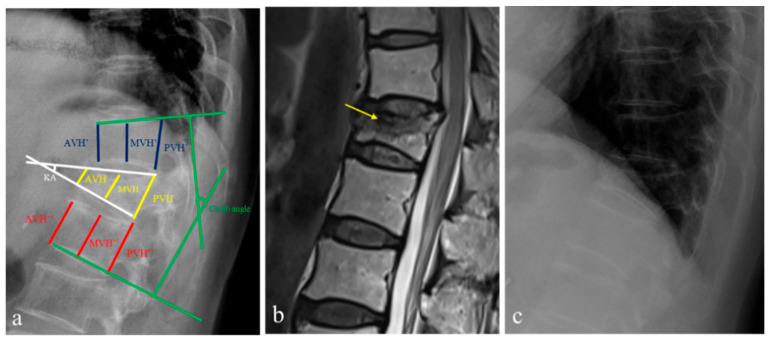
A 60-year-old woman who was diagnosed with an osteoporotic vertebral fracture (OVF) at T12. (a) To investigate morphological parameters, the kyphotic angle (KA), Cobb angle, anterior vertical height (AVH), middle vertical height (MVH), and posterior vertebral height (PVH) along with the AVH, MVH, and PVH of the adjacent upper and lower levels (AVH’ and AVH’’, MVH’ and MVH’’, PVH’ and PVH’’) were measured on lateral radiographs. From these parameters, the vertebral body compression ratio (VBCR), the percentage of anterior height compression ratio (PAHC), and the percentage of middle height compression ratio (PMHC) were calculated. (b) Sagittal magnetic resonance imaging showed an endplate type of T12 OVF with the presence of an intravertebral cleft sign (yellow arrow). (c) After two-years of follow-up with zoledronic acid medication, the VBCR, PAHC, and PMHC in lateral radiographs were also measured for the assessment of morphological factors.
